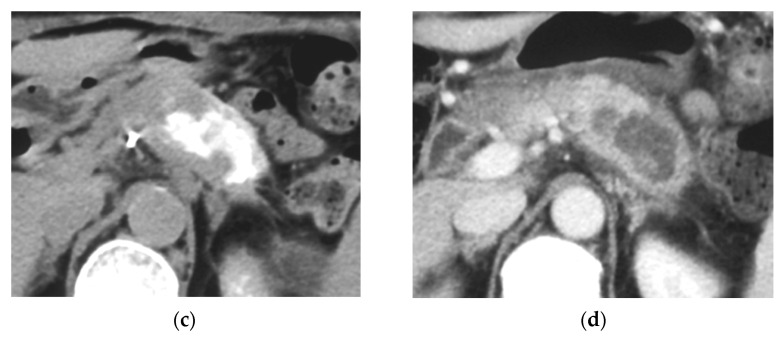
Pilot study. A 66-year-old female had locally advanced pancreatic carcinoma at body and tail. An arterial administration of DNA crosslinking agents achieved good tumor necrosis. Patient had died after 12 months. Dense retention (dense contrast materials in area >50% of tumor). (a) Postcontrast CT shows a mass at the pancreatic tail. A hypodense mass occupies pancreas body and tail encasing splenic vein (arrow). (b) Superselective dorsal pancreatic angiography shows severe encasement of the transverse pancreatic artery. Arrows indicate the presence of a hypovascular mass. (c) CT immediately taken after administration of the drugs mixed with contrast media shows dense contrast retention in area >50% of tumor. (d) Follow-up CT two weeks after the superselective infusion shows massive necrosis in the tumor.
